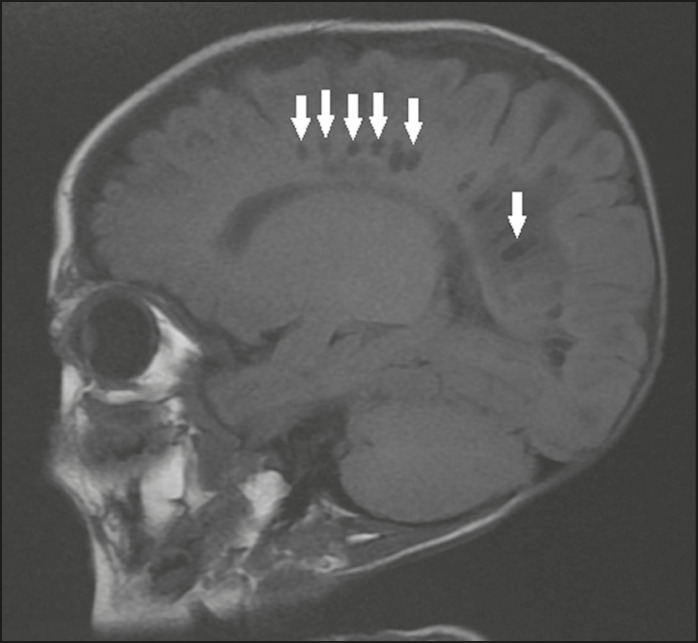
Mucopolysaccharidosis. T1-weighted sagittal MRI showing multiple cystic images with a signal similar to CSF, affecting the region around the corpus callosum (arrows), resulting in enlarged perivascular spaces.
